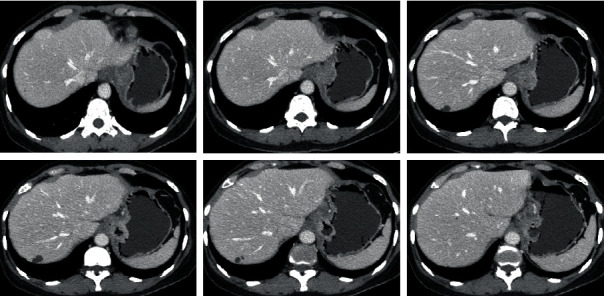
CT images of gastric cancer (female patient, 45 years old).
